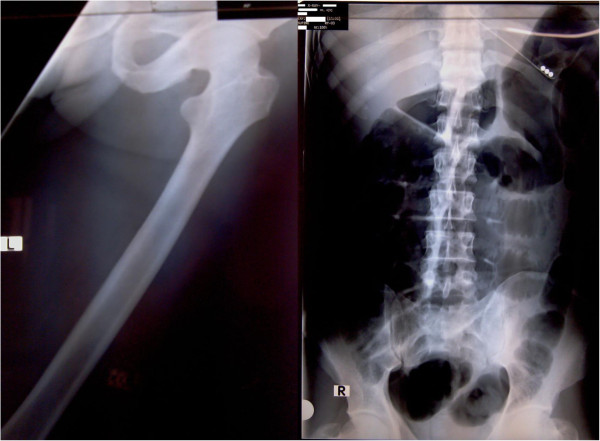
X-ray imaging of left thigh and abdomen. Image on the left is an X-ray of the left thigh; it failed to demonstrate presence of gas. Image on the right is an X-ray of the abdomen in supine position which demonstrates dilated large bowel loops favoring the clinical picture of intestinal obstruction.
